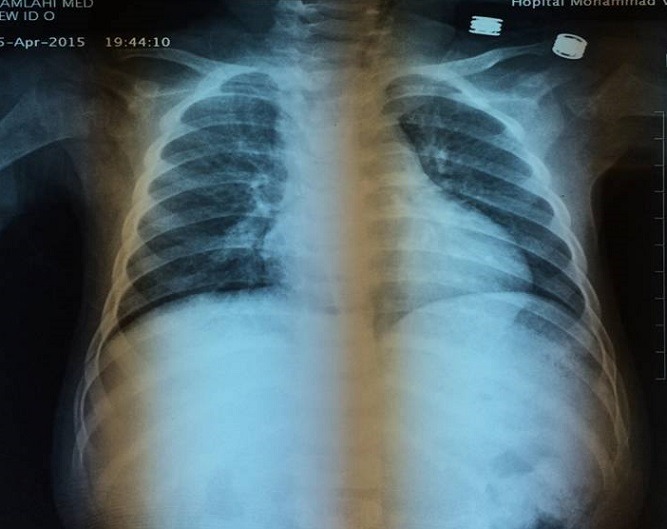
Radiographie thoracique montrant une déviation trachéale à gauche avec un index cardiothoracique normale
